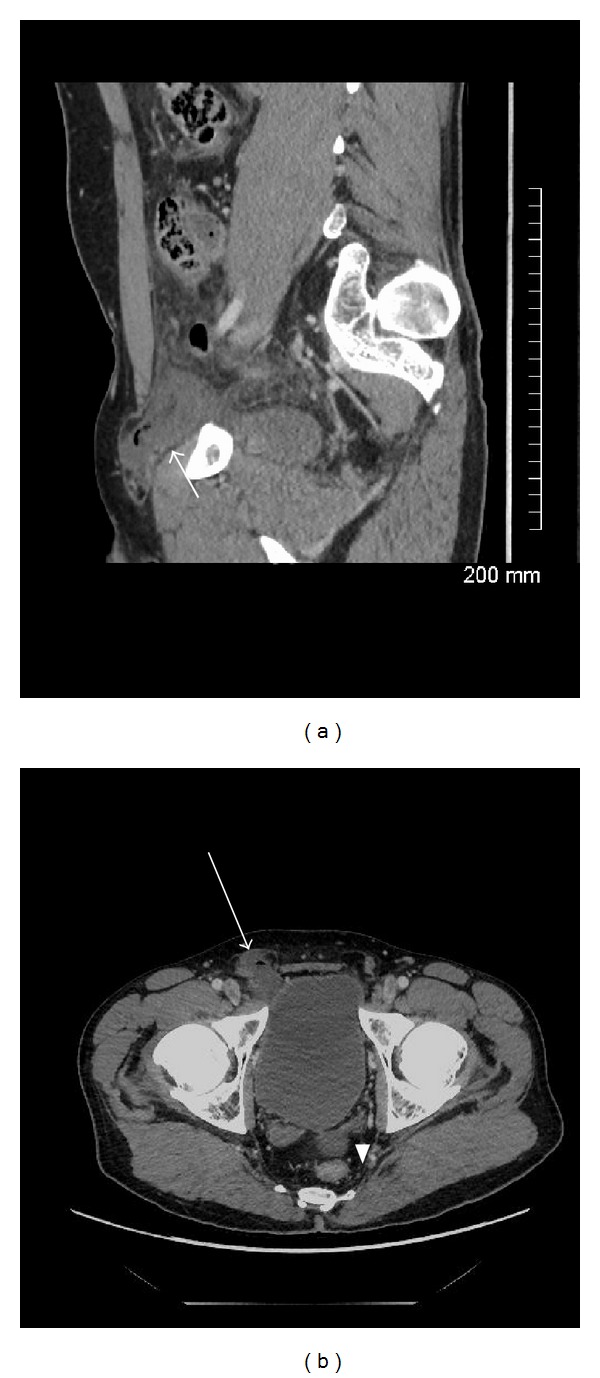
Contrast enhanced coronal (a) and axial (b) CT of the abdomen in a 45-year-old male with an inguinal trauma, showing right inguinal hernia, with extraluminal air (white arrow) and fluid in the peritoneal cavity (arrowhead).
